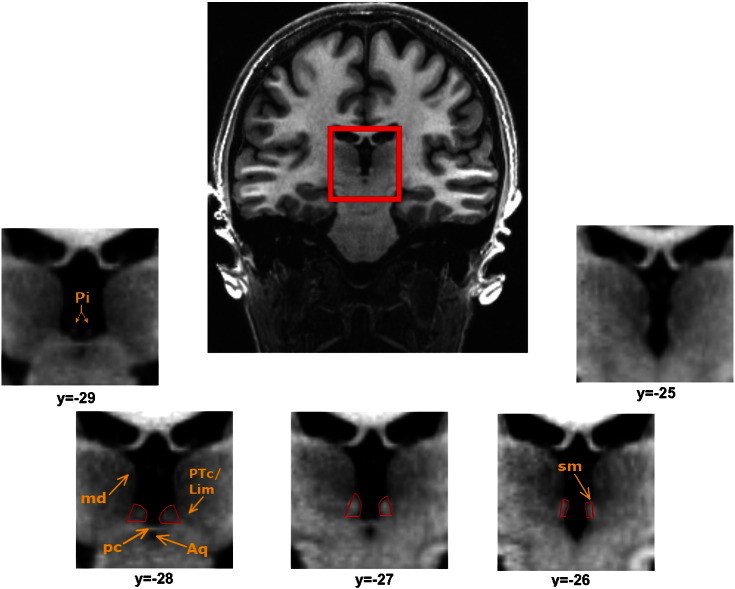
Coronal MRI sections of the habenula (outlined in red) and neighbouring anatomical landmarks on T1-weighted MRI images. The habenula appears brighter than the adjacent mediodorsal thalamic nucleus due to relatively dense white matter fibres. In the more posterior planes the habenula protrudes into the third ventricle and appears pyramidal in shape; moving more anterior, the habenula shifts slightly dorsally and appears more oblong in shape. An individual representative subject is shown. Note that the y co-ordinate of the PC will vary across individuals. Aq = cerebral aqueduct, pc = posterior commissure, Pi = pineal stalk, md = mediodorsal thalamic nucleus, lim/pc = limitans nucleus/pretectal area, sm = stria medullaris.
